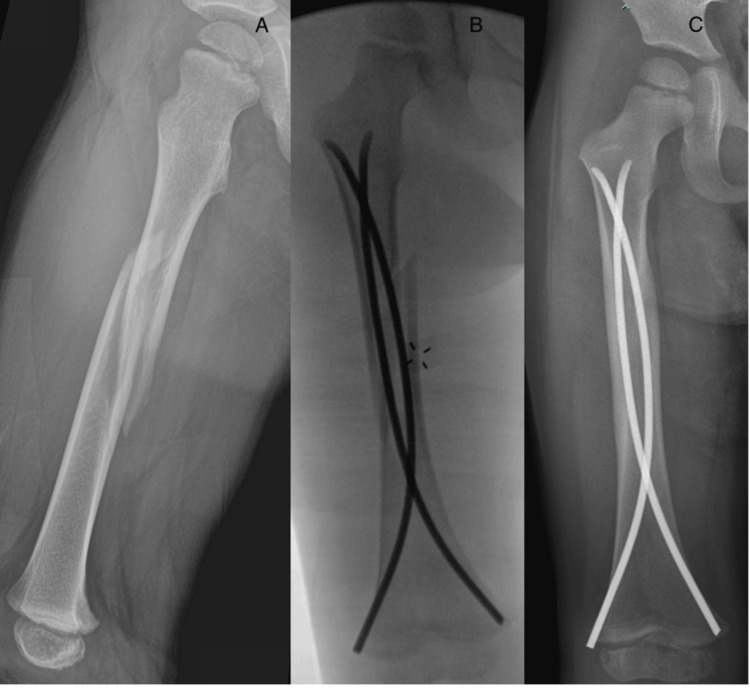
Original perioperative images of titanium elastic nail fixation. A 3.5-year-old boy with a right femoral shaft fracture (A). Intraoperative image showing the fracture fixation using two titanium elastic nails (B). An X-ray image taken four months after surgery showing a well-aligned femoral shaft (C).
